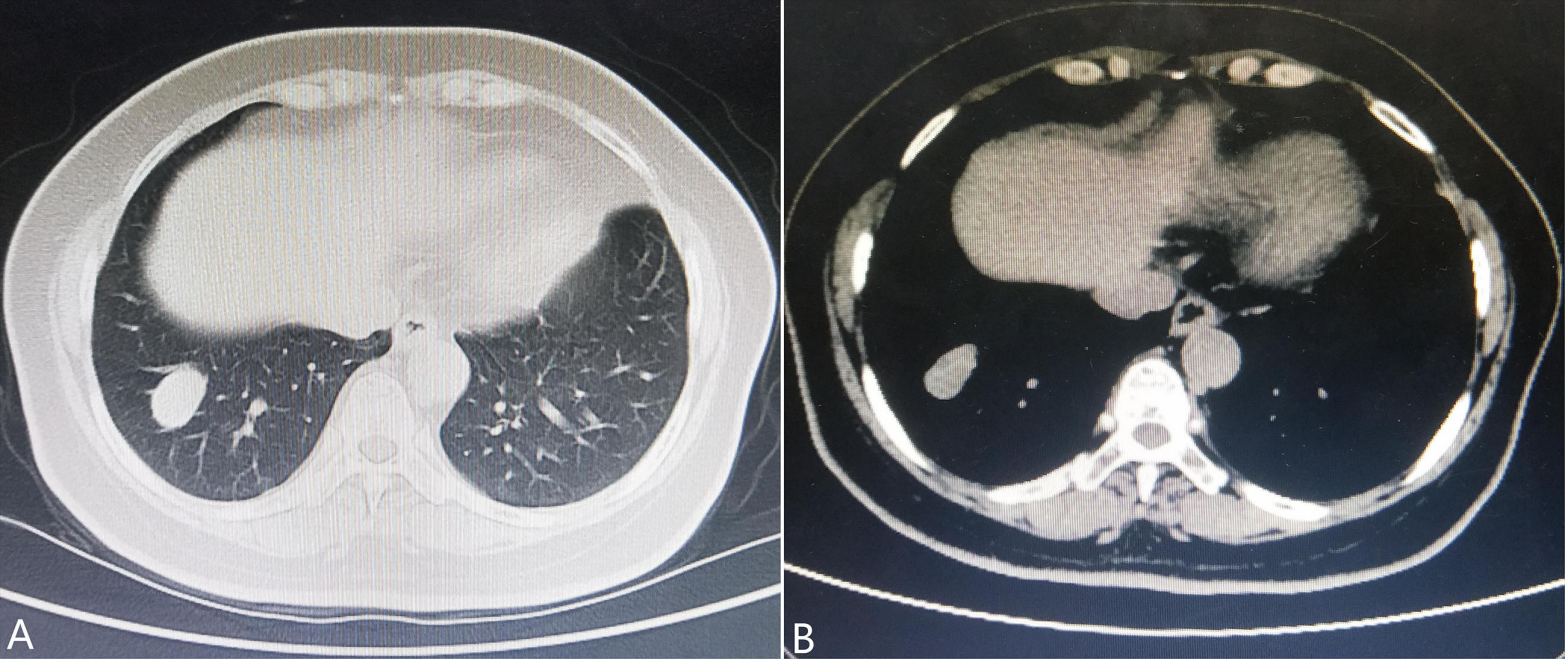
Chest computed tomography shows (A) a nodulous shadow located in the segmentum basale laterale of the right lower lobe measuring 2.6 cm × 1.8 cm in size with a smooth margin and (B) soft tissue density.
